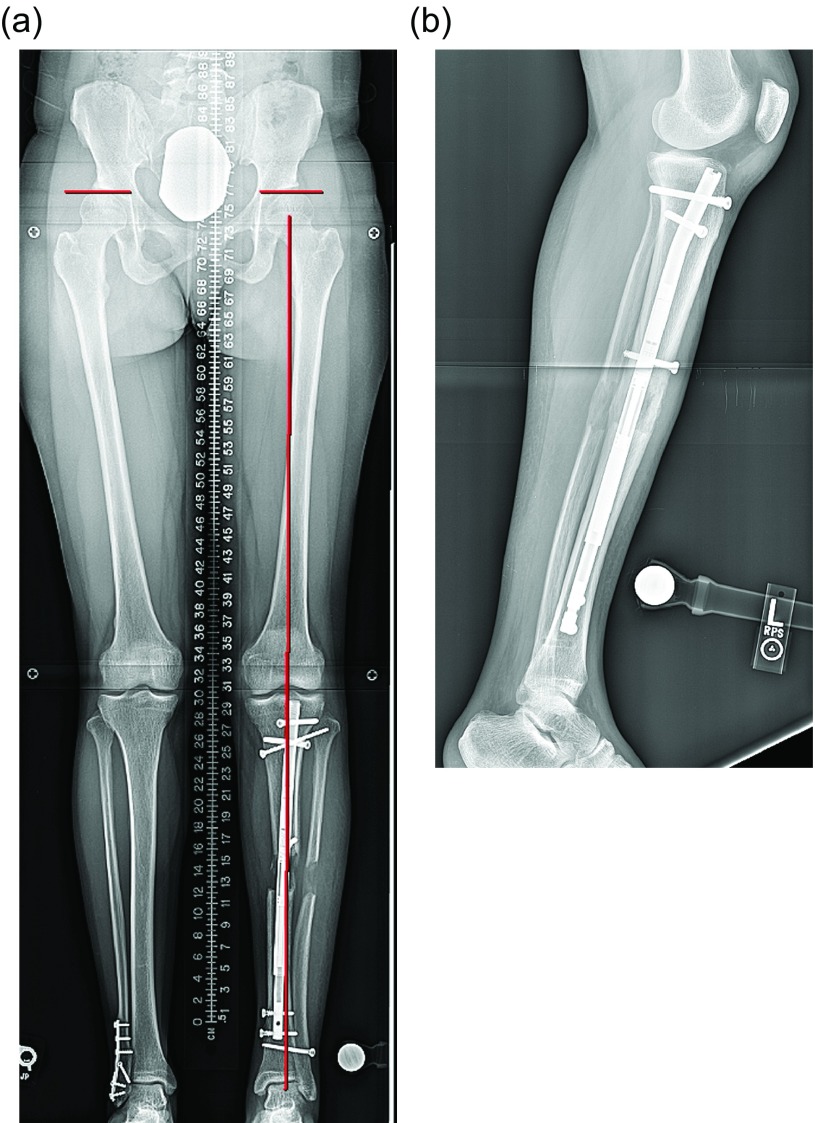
(a) The X-rays shown in Figures 15a and 15b are a continuation of the patient presented in Figures 10a and 10b. The end of distraction long-standing radiograph shows equal limb length and ideal alignment. (b) A lateral radiograph shows interval healing of the regenerate and ideal sagittal alignment.
